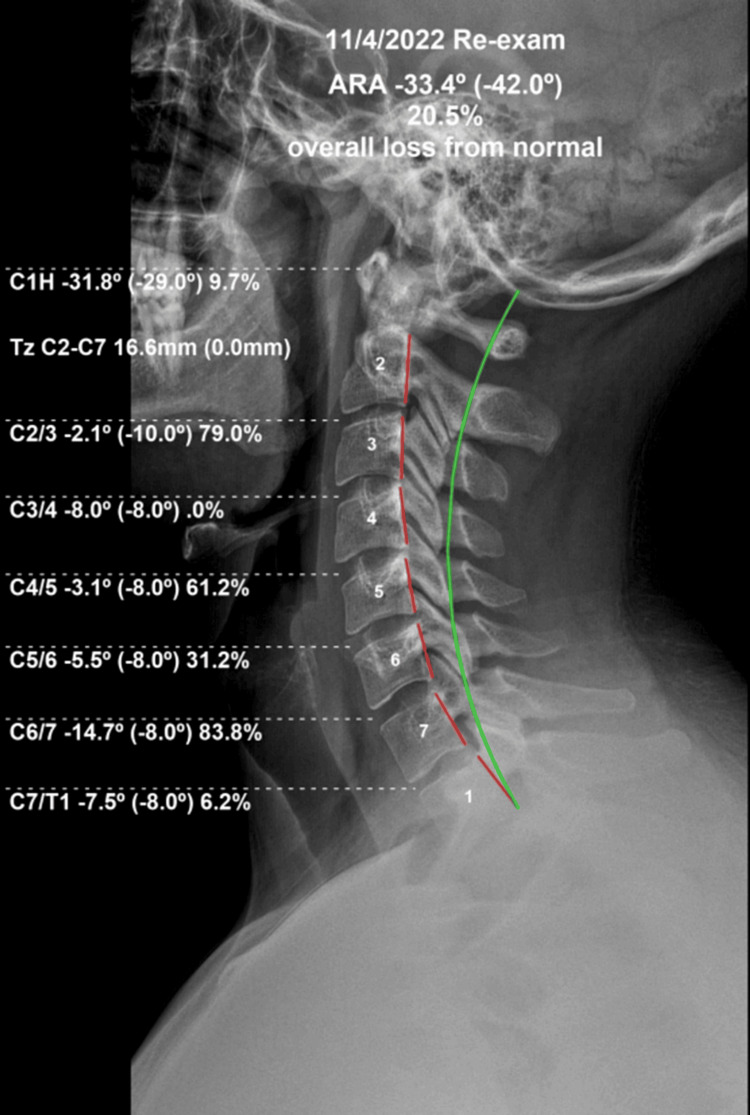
Re-evaluation upright lateral cervical radiograph Following 24 treatments in-office with home therapies according to CBP® protocols, a much improved deep cervical lordosis is visualized. The green line represents an ideal lordosis, and the red dashed line measures the posterior tangents of the segments. Values within parentheses are ideal, and values outside the parentheses represent the patient's parameters as measured with PostureRay®
